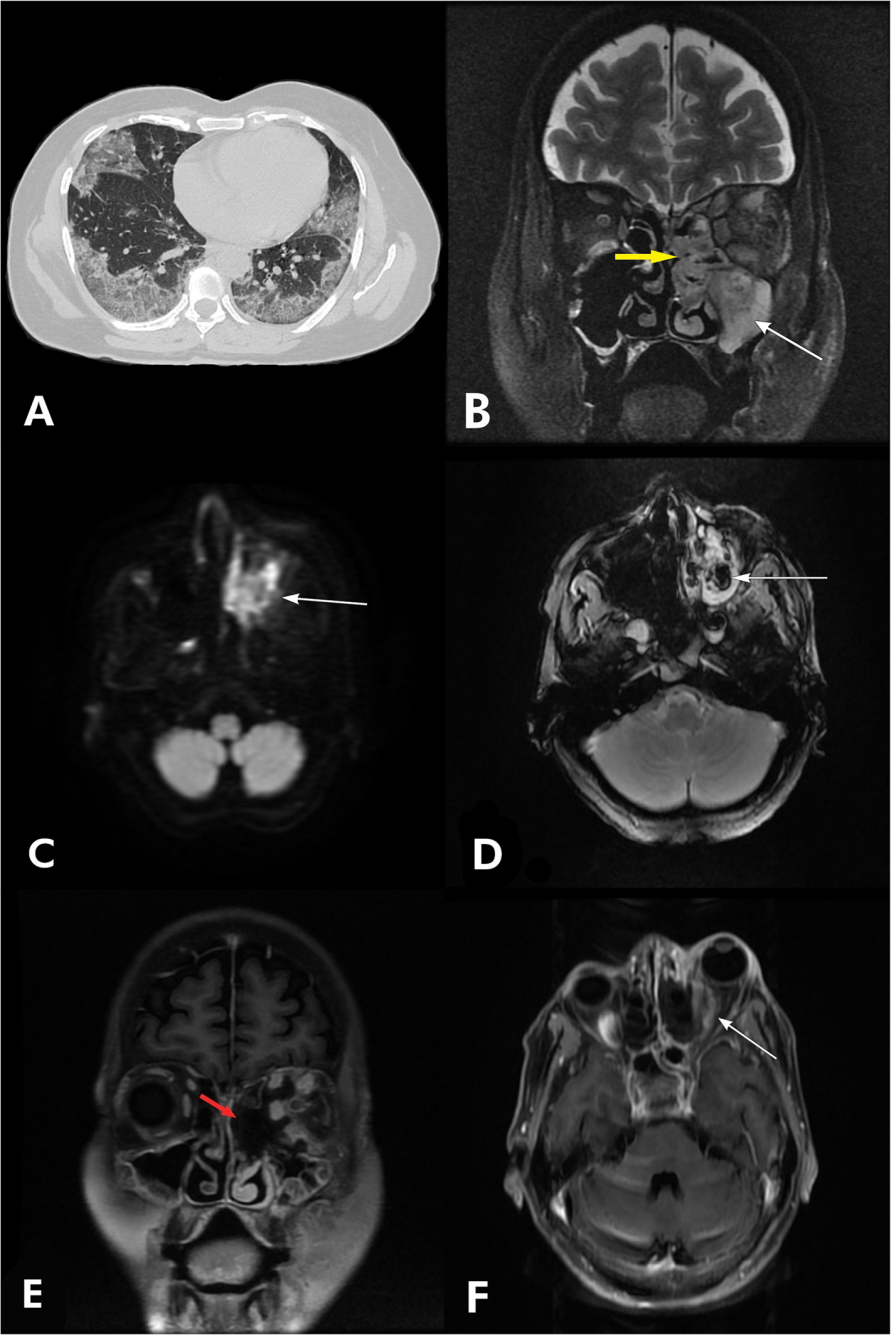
a Axial HRCT thorax image showing ground-glass opacities in subpleural regions of bilateral lung parenchyma with “crazy paving appearance.” b Coronal T2 FS image showing mucosal thickening and collection in the left maxillary sinus (white arrow), left ethmoidal sinus, and left middle nasal turbinate (yellow arrow) with inflamed extraocular muscles. c Axial DWI image showing restricted diffusion in the left maxillary sinus and left middle nasal turbinate. d Axial GRE image showing foci of blooming in the left maxillary sinus (white arrow). e Coronal T1 post-contrast image showing area of non-enhancing soft tissue in left middle nasal turbinate and within the left maxillary antrum (“black turbinate sign”). f Axial T1 post-contrast image showing enhancement and inflammation involving extraocular muscles of left orbit causing proptosis
